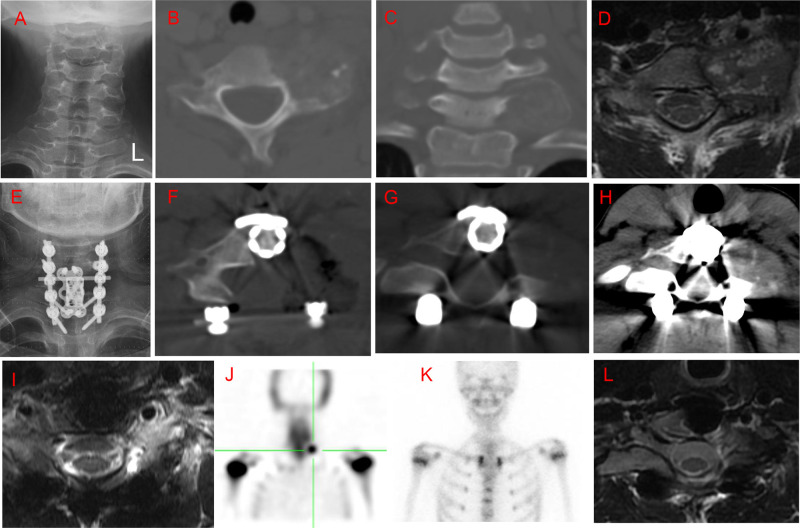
Case 5. A 15-year-old male patient with local neck and night pain for 6 months. An osteolytic lesion was located in the C7 vertebral body, left pedicle, transverse process, and lateral mass, with left vertebral artery extension. He underwent intralesional marginal resection; however, positron emission tomography-computed tomography (PET-CT) 7 months postoperatively showed local recurrence. The patient underwent a second surgery through a posterior approach. There was no further recurrence at a 5-year follow up after the second surgery. (A) Preoperative posteroanterior radiography. (B,C) Preoperative axial and coronal computed tomography (CT) scans. (D): T2-weighted magnetic resonance imaging (MRI) scan. (E) Posteroanterior radiography 1 week after the first surgery. (F). Axial CT scan 1 week after surgery. (G,H) Axial CT scan 7 months after surgery. (I) T2-weighted MRI scan 7 months after surgery. (J) Isotope bone scan 7 months after surgery. (K) Isotope bone scan 3 years after the second surgery. (L) T2-weighted MRI scan 5 years after the second surgery.
